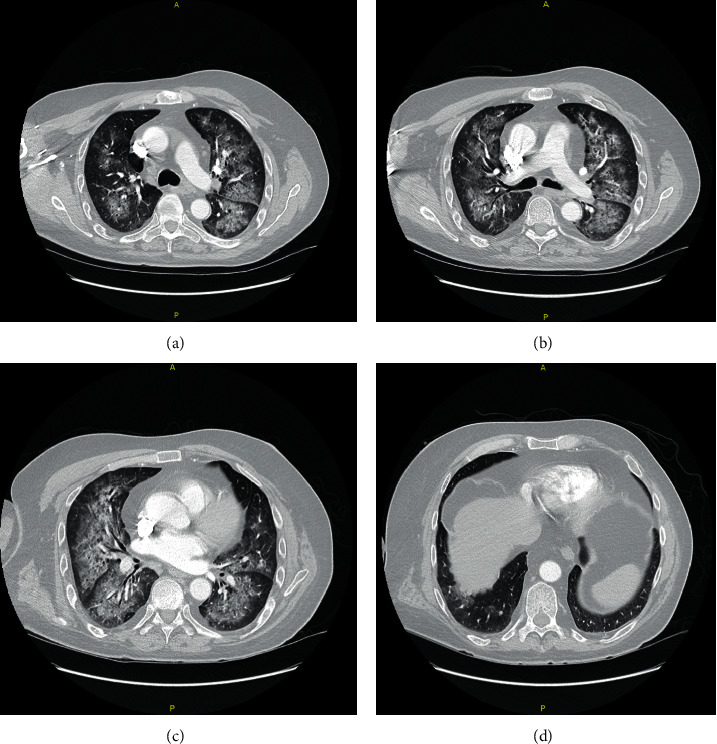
Extensive patchy airspace disease on CT scan of the chest with contrast.
